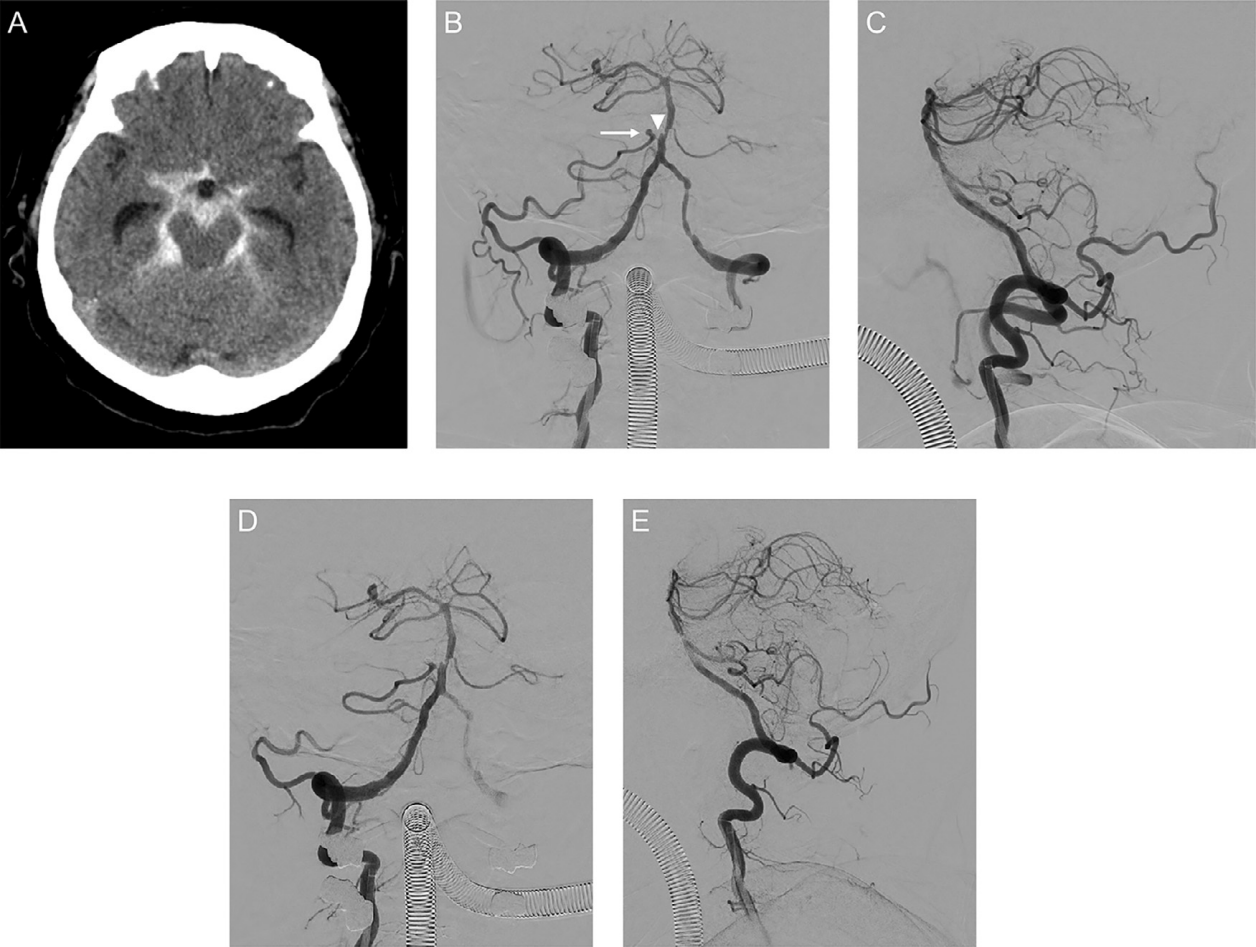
(A) Computed tomography showing subarachnoid hemorrhage. Posteroanterior (B) and lateral (C) views of left vertebral digital subtraction angiography showing a 3 mm aneurysm of the anterior inferior cerebellar artery along an arterial bend (arrow). Stenosis of the proximal parent artery and the basilar artery are also apparent (arrow heads). The ipsilateral posterior inferior cerebellar artery is hypoplastic (dotted arrow). Posteroanterior (D) and lateral (E) views of left vertebral digital subtraction angiography after coil embolization showing occlusion of the aneurysm and preservation of the parent artery.
